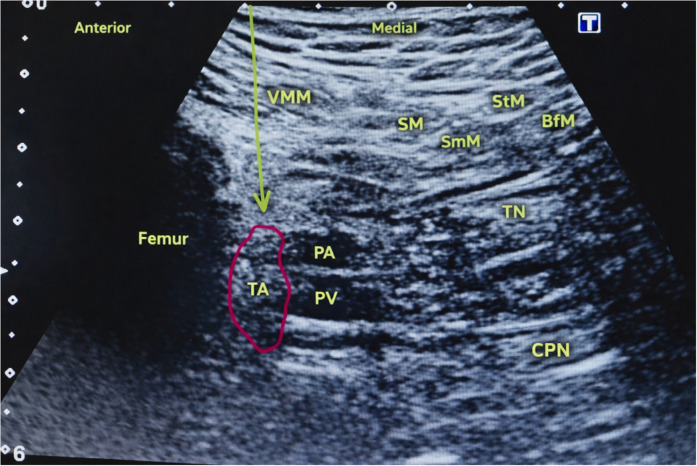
Ultrasound-guided IPACK block demonstrating the Target area (TA) and surrounding anatomical structures. PA: Popliteal artery, PV: Popliteal vein, TN: Tibial nerve, CPN: Common peroneal nerve, VMM: Vastus medialis muscle, SM: Sartorius muscle, StM: Semitendinosus muscle, SmM: Semimembranosus muscle, BfM: Biceps femoris muscle
